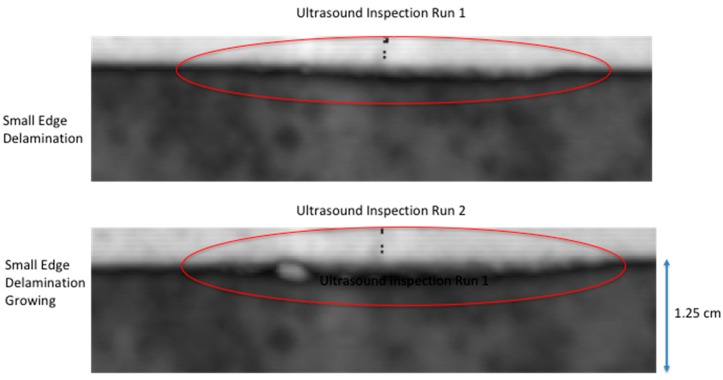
Ultrasonic inspection image after quasi-static second load run with comparison to initial run ultrasonic inspection.
